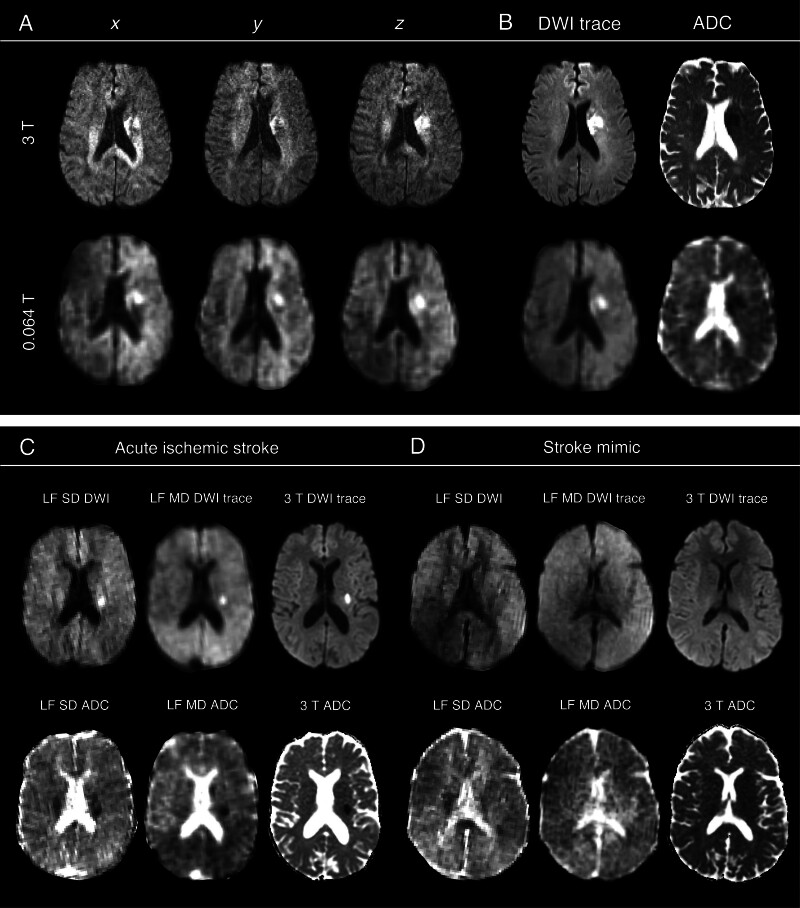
Comparison of individual diffusion directions at 0.064 T (v1 system) and 3 T field strengths against averaged trace diffusion-weighted imaging (DWI) and apparent diffusion coefficient (ADC) counterparts. A, Individual diffusion directions (*x*, *y*, and *z*) demonstrate variable lesion conspicuity at both field strengths. B, In comparison, averaged trace DWI and ADC counterparts show improved signal uniformity, whereby signal from anisotropically restricted white matter tracts is attenuated, enabling improved detection of isotropically restricted diffusion in ischemic cells. C, A case example from a patient with an acute ischemic lesion in the left corona radiata, which can more readily be seen on the 0.064 T multi-direction (MD) ADC map compared with the single-direction (SD), where corticospinal tracts obfuscate the lesion. D, A case example from a patient with a stroke mimic, where greater signal variability is seen on the SD 0.064 T DWI and ADC compared with MD counterparts. No diffusion abnormality can be seen on the conventional 3 T DWI nor ADC. The skull has been stripped for visualization purposes. Each image shown at 0.064 T is from v1 hardware. LF indicates low-field.
